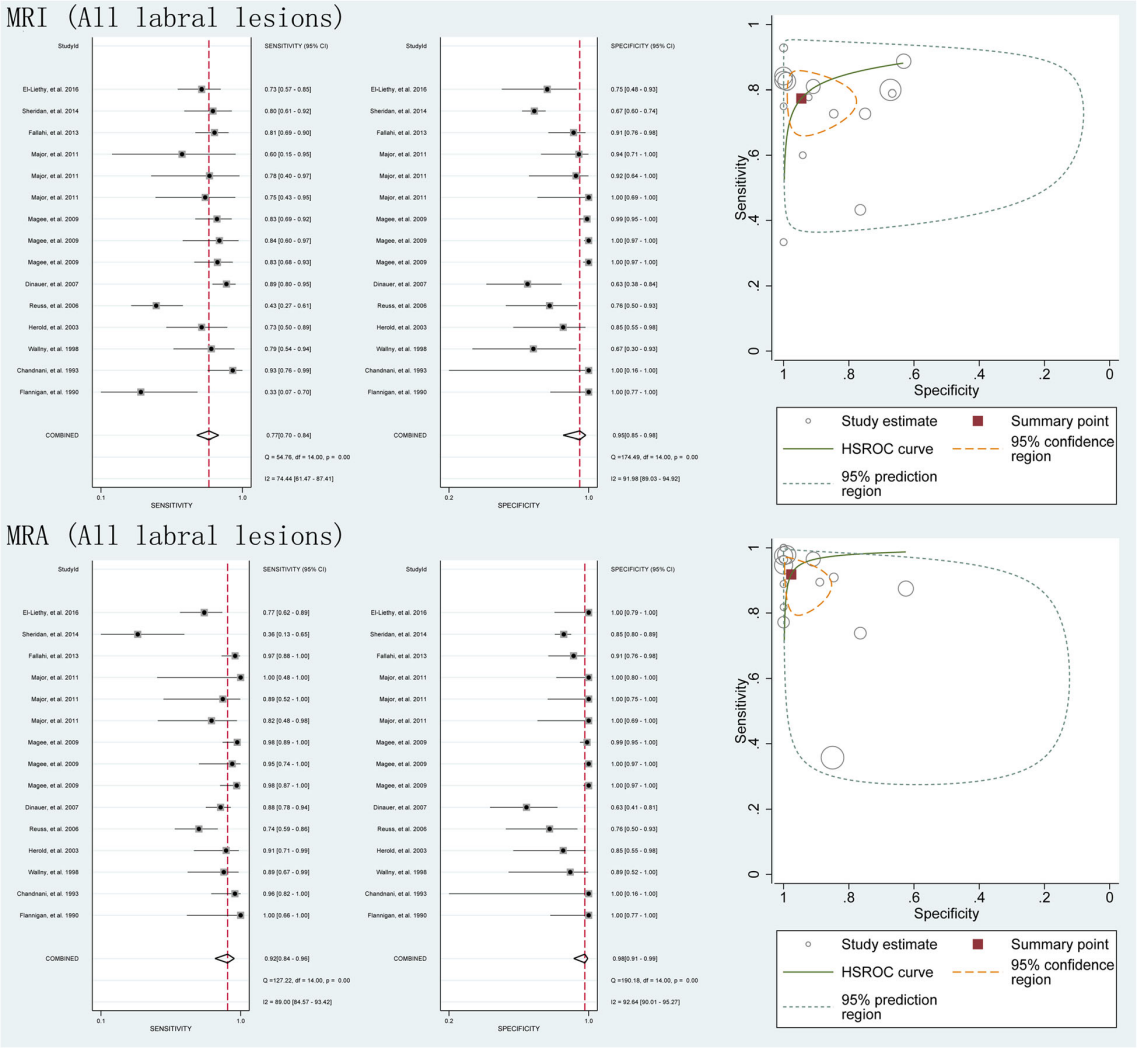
Pooled sensitivity, specificity and HSROC of MRI and MRA for detecting all labral lesions
